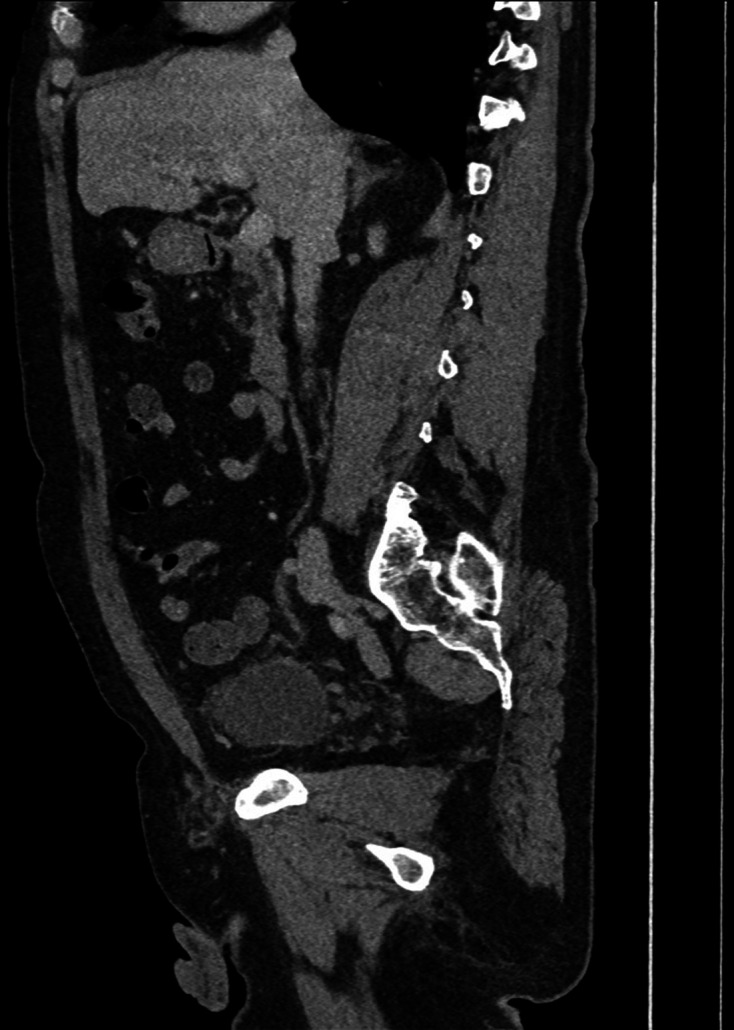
Third coronal delayed phase CT (3 months following admission), on soft tissue
